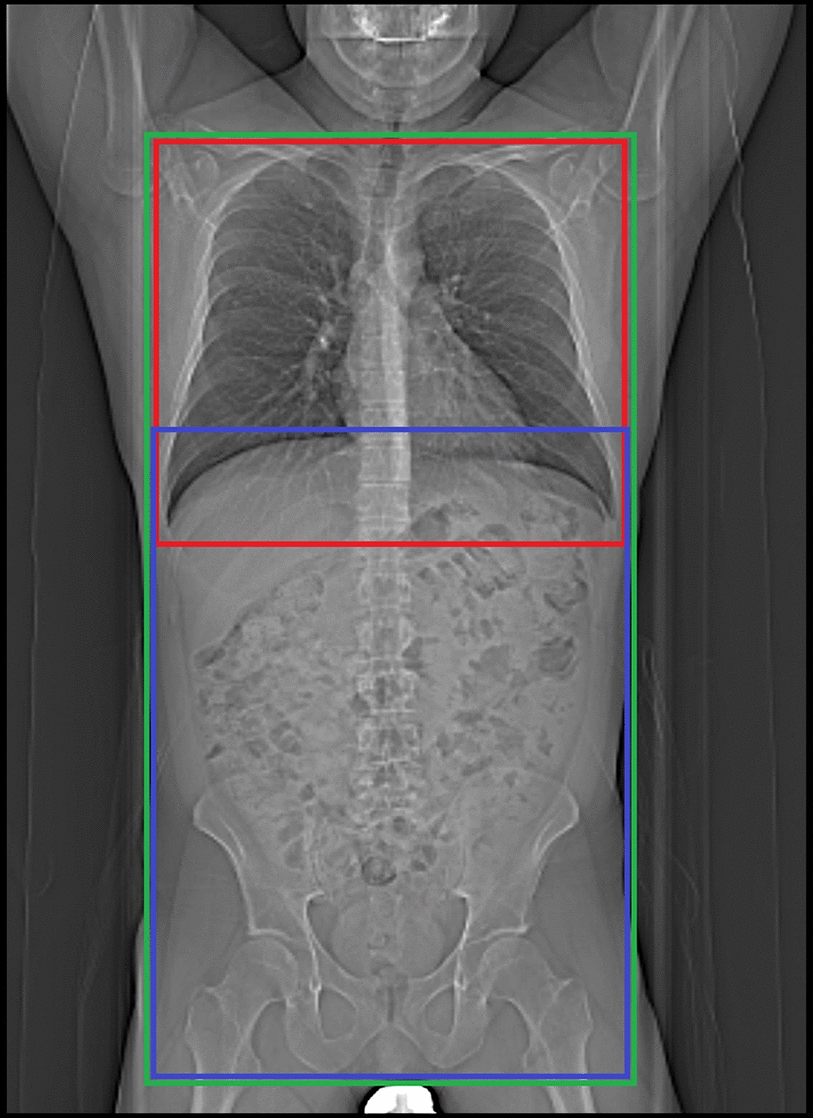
CT planning scout. Green: scout for thoracoabdominal CT. Red: examined region in thoracic CT alone. Blue: examined region in abdominal CT alone
